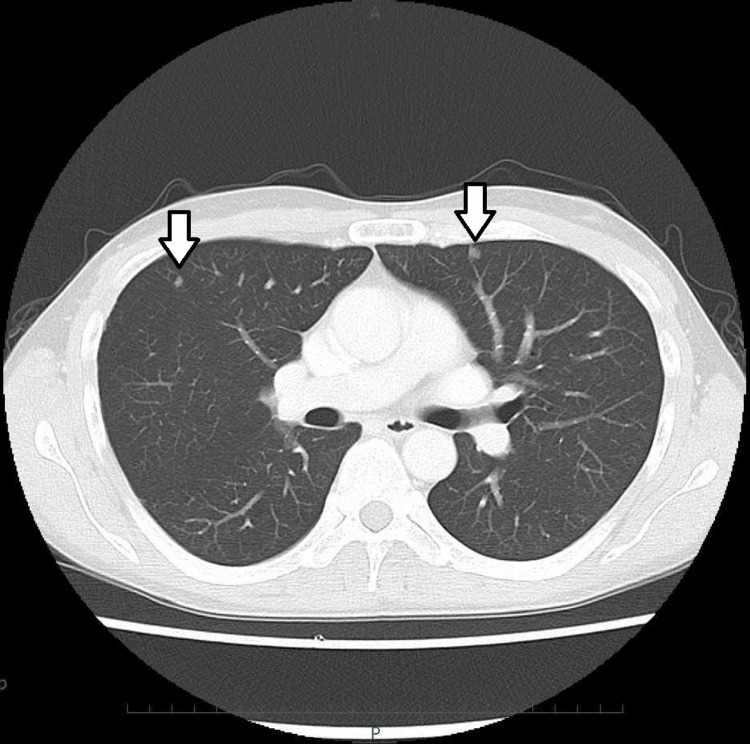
CT scan prior to EV treatment showing two lesions in the lung (white arrows). EV: enfortumab vedotin
